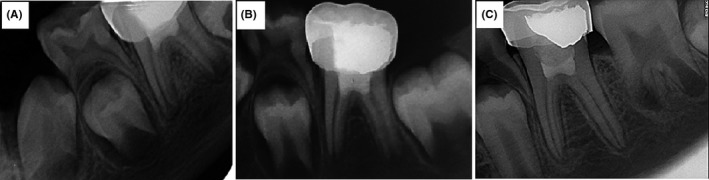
Follow‐up radiographs after pulpotomy. (A) 3‐mo follow‐up, (B) 9‐mo follow‐up, and (C) 24‐mo follow‐up which show complete root development and closed apex
